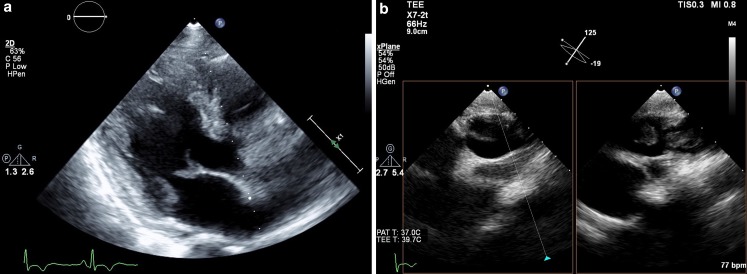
Transthoracic (a) and transoesophageal echocardiography (b) revealing a mobile structure attached to the ventricular side of the anterior mitral valve leaflet and multiple mobile masses at the origin of the pulmonary artery
